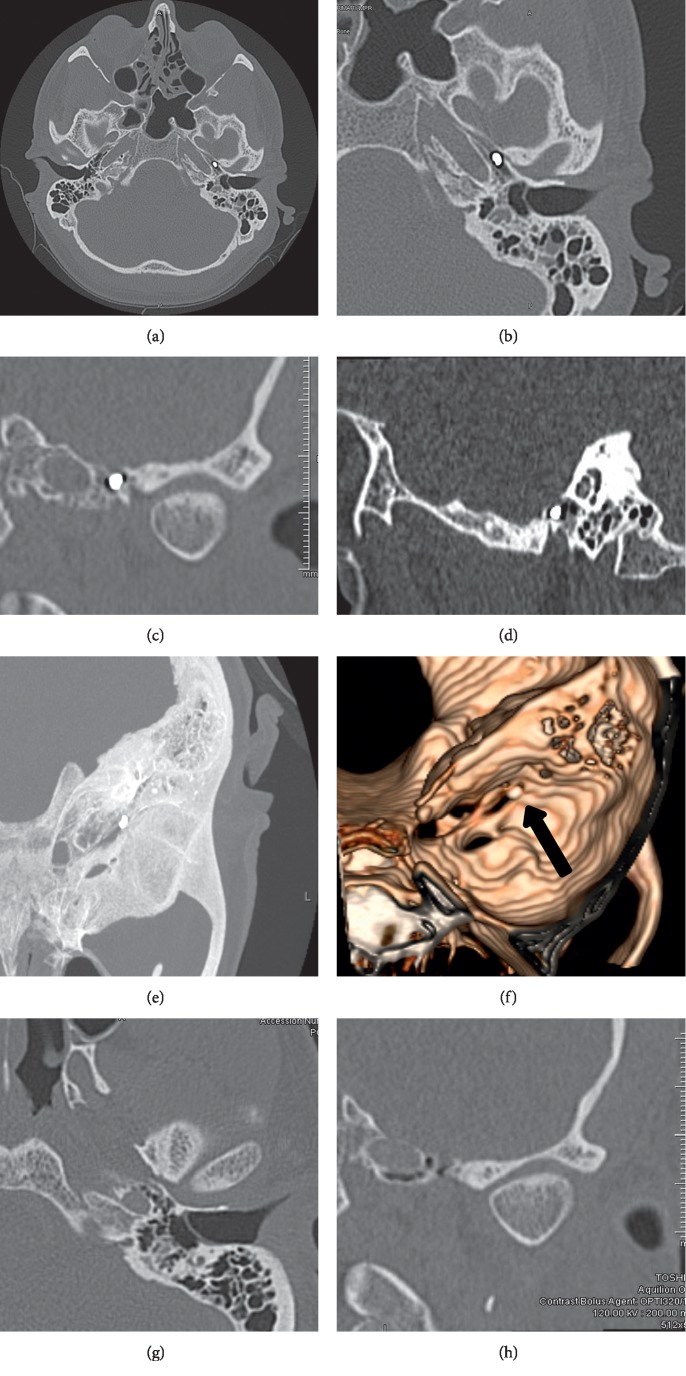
Coronal, axial, and sagittal CTs demonstrating radiopaque foreign body at the level of the bony eustachian tube. (a) Axial CT demonstrating foreign body in the left eustachian tube with normal appearance of the right eustachian tube. (b) Magnified axial CT also demonstrating the proximity to the carotid artery. (c) Coronal CT with object in the left bony eustachian tube. (d) Sagittal CT. (e) Oblique MIP in the same plane as the 3D reconstruction. (f) 3D reconstruction with arrow to the foreign body. (g) Postoperative axial CT. (h) Postoperative coronal CT.
